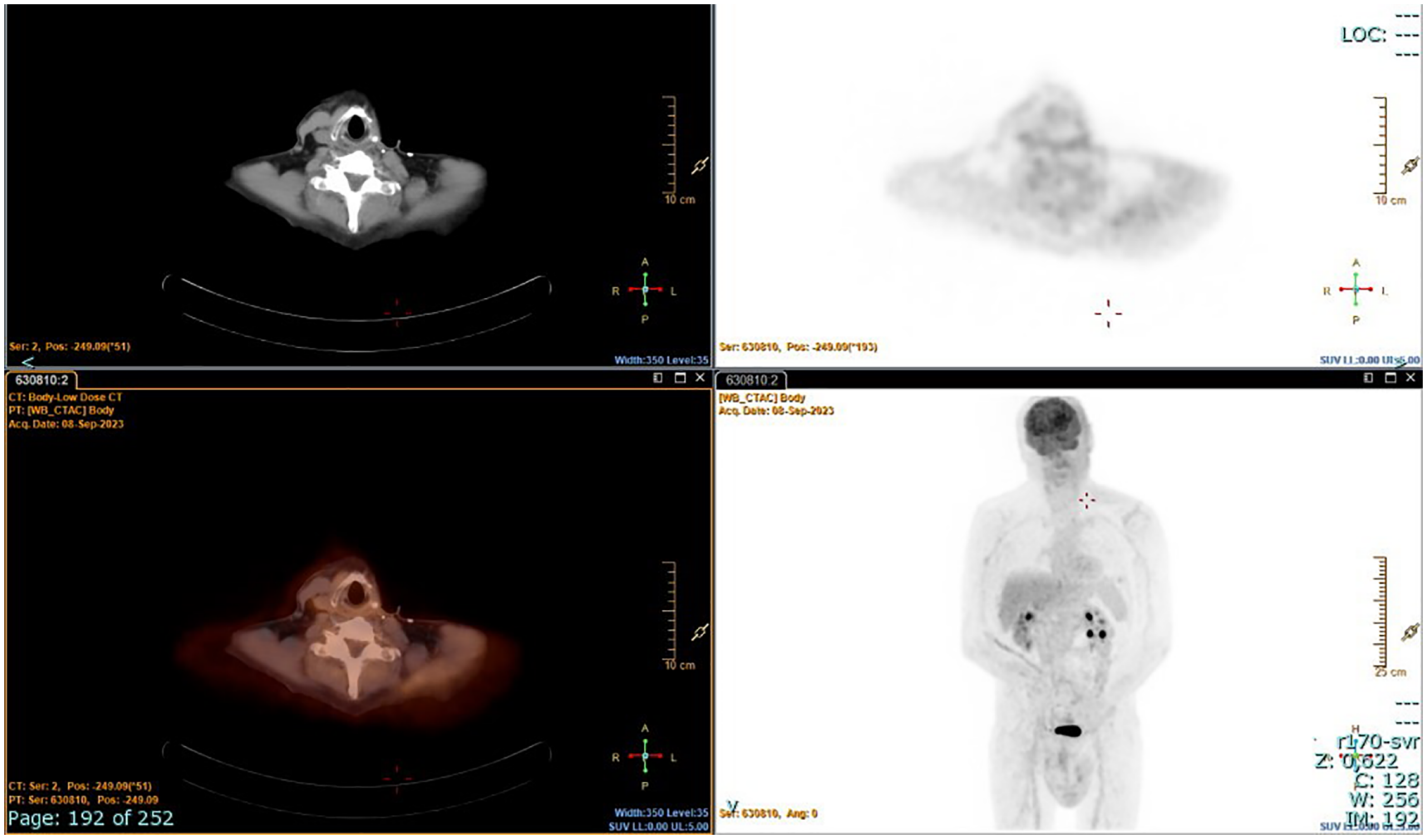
^18^FDG/PET-CT scan after 12 months. No pathological uptake.
